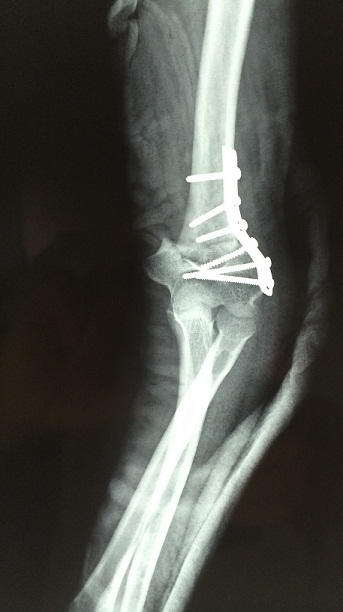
Radiographie de face montrant une fixation par plaque vissée, l'ostéosynthèse est restée stable même si l'ostéotomie a entamé la corticale interne
